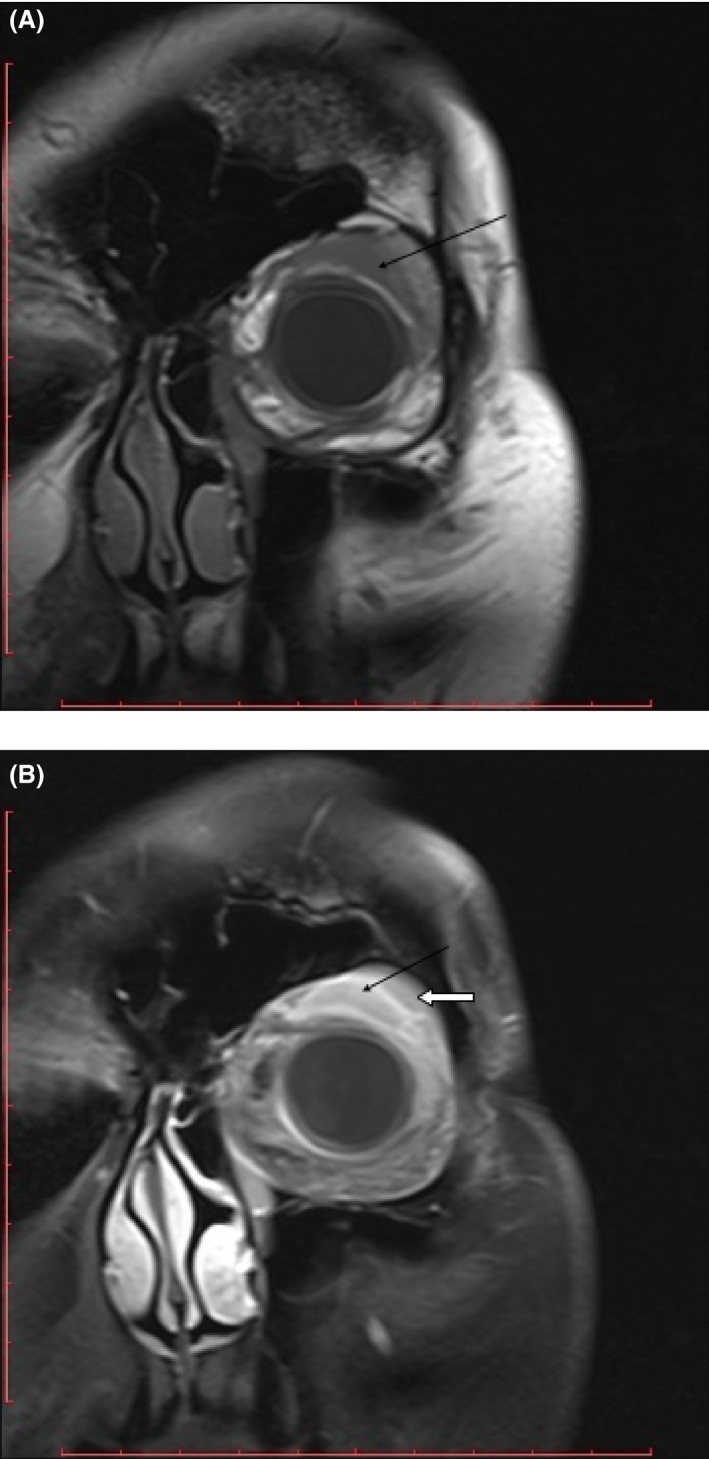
(A) MRI T1 TSE, coronal plane (without contrast). (B) MRI T1 TSE FS, coronal plane (with contrast). Black arrow – pathologic mass; White arrow – lacrimal gland.
